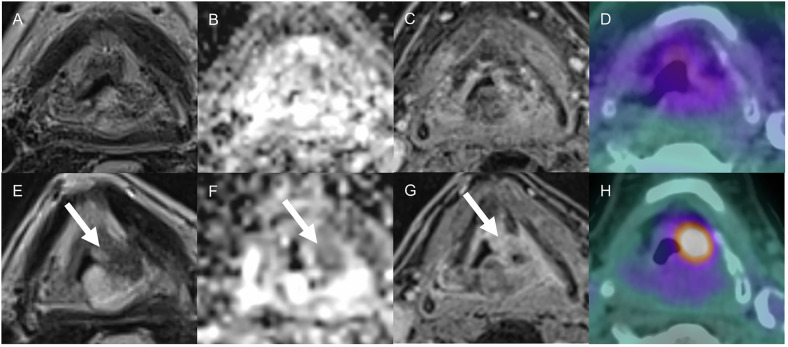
59-year-old man with a history of c4N1 oropharynx squamous cell carcinoma, positive for human papillomavirus after chemo and radiotherapy. Axial T2 TSE sequence (A), ADC map (B), T1 sequence after contrast injection (C), and FDG-PET/CT (D) showing negative follow-up examinations. MRI obtained 3 years post-treatment (E–G) demonstrates a nodule at the primary site (arrows) with intense focal FDG uptake (H). Primary site was assigned to the NI-RADS 3 category. Biopsy was performed and confirmed recurrence
